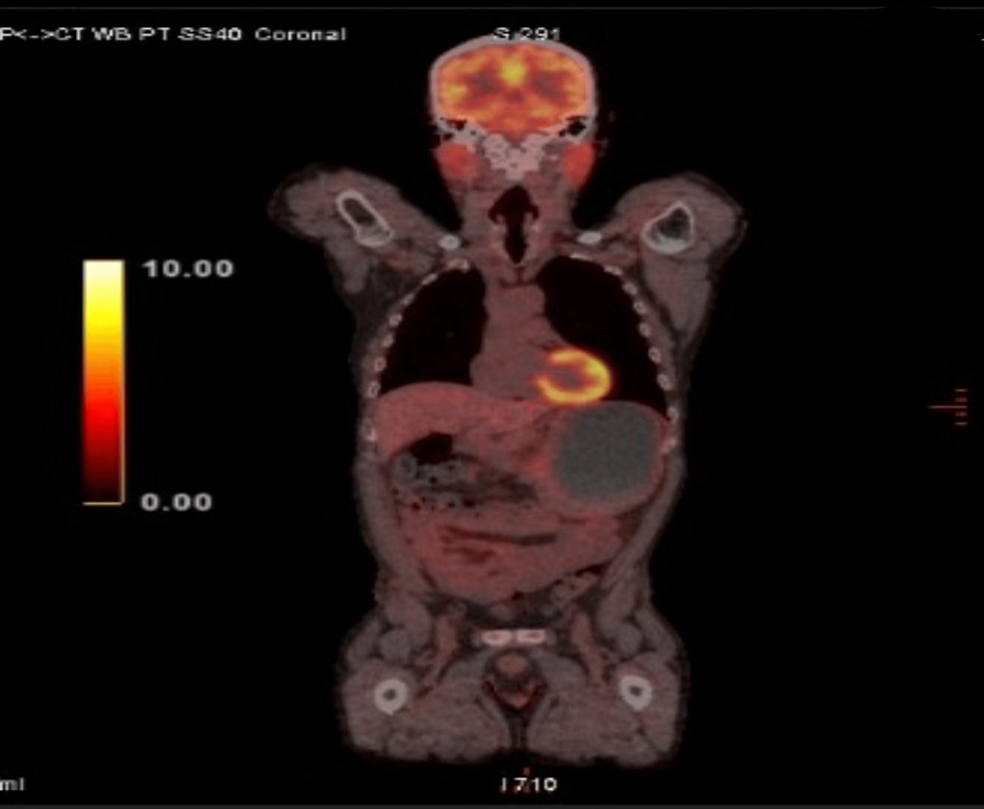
Positron emission tomography-computed tomography scan showing a complete regression of the adenopathy. This image was obtained three months after the previous scan shown in Figure 4.
